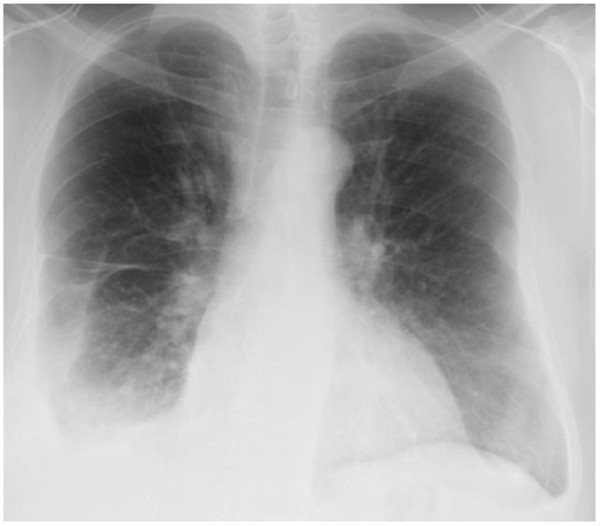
Chest x-ray at 1 month after discharge: Significant right lung re-expansion.
